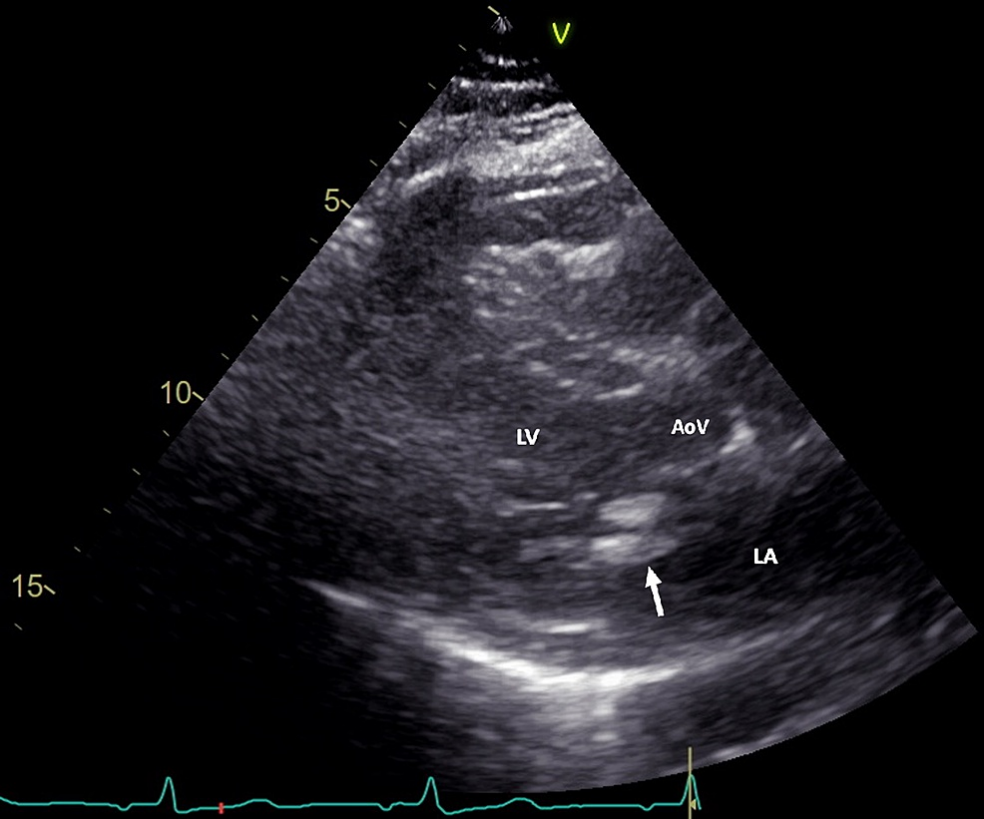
Transthoracic echocardiogram showing a parasternal long-axis view image of the heart. The white arrow shows the large calcified mass attached to the posterior aspect of the mitral valve. LA = left atrium, LV= left ventricle, AoV = aortic valve.
